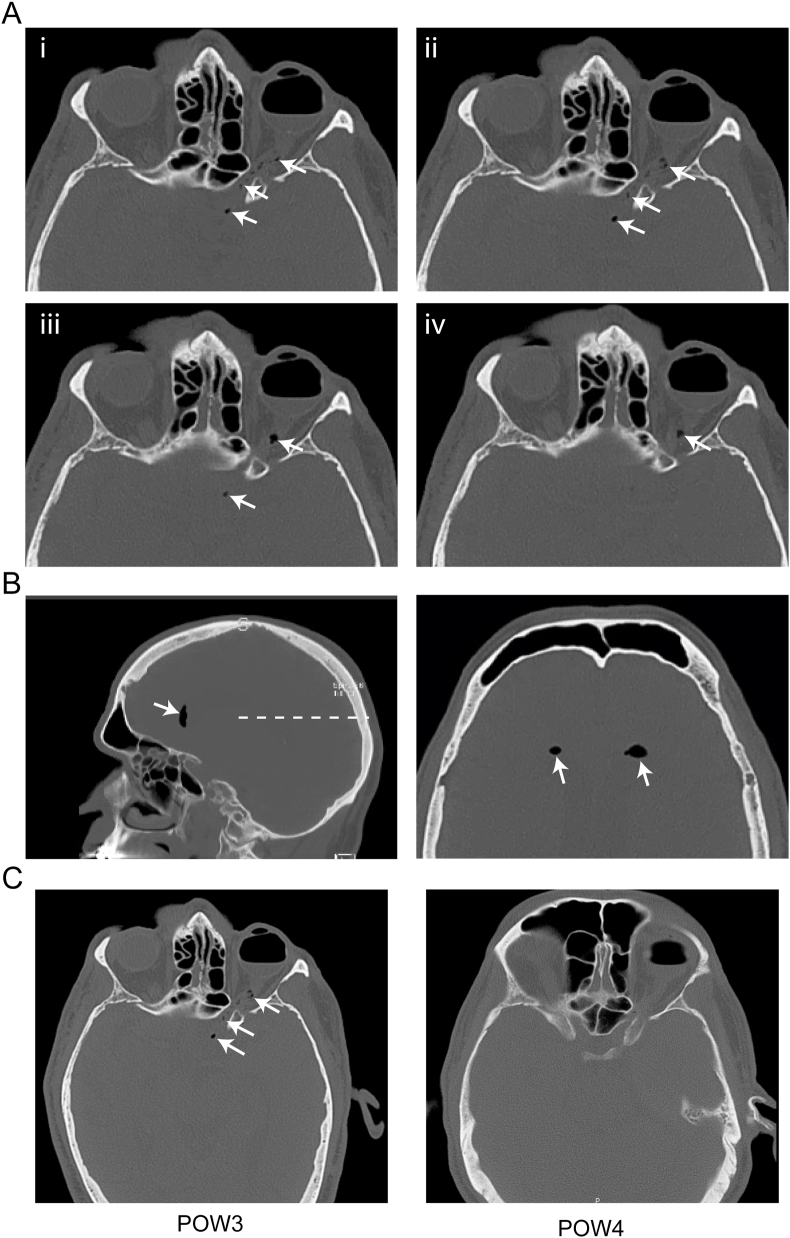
Intracranial gas migration and subsequent resolution. (A) Inferior-to-superior axial series from a non-contrast CT scan of the head at post-operative week 3 shows about a 60% gas fill in the vitreous cavity of the left eye (i-iv). Radiolucent spaces are seen infiltrating the intraorbital, canalicular, and prechiasmatic segments of the optic nerve (white arrows), consistent with intracranial extension of gas. (B) Sagittal view of the same CT scan in (A) shows pneumocephalus in the anterior horn of the lateral ventricle (left, white arrows). An axial view at the level of the dashed line in the left image demonstrates bilateral pneumocephalus (right, white arrows). (C) An axial image from the same CT scan at post-operative week 3 (POW3) as in (A) shows radiolucent spaces in the optic nerve (left, white arrows). Follow up non-contrast axial CT scan one week later (POW4) shows about a 50% intraocular gas fill with resolution of the previously-observed radiolucent spaces along the optic nerve and within the ventricles (right). These CT images were taken from a similar plane at the level of the optic nerve, however other cranial structures are out-of-plane due to differences in head positioning.
